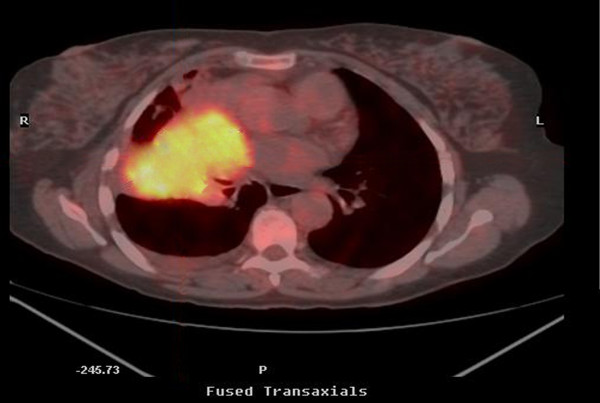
Axial fused PET-CT demonstrating FDG accumulation in a right middle lobe mass.
